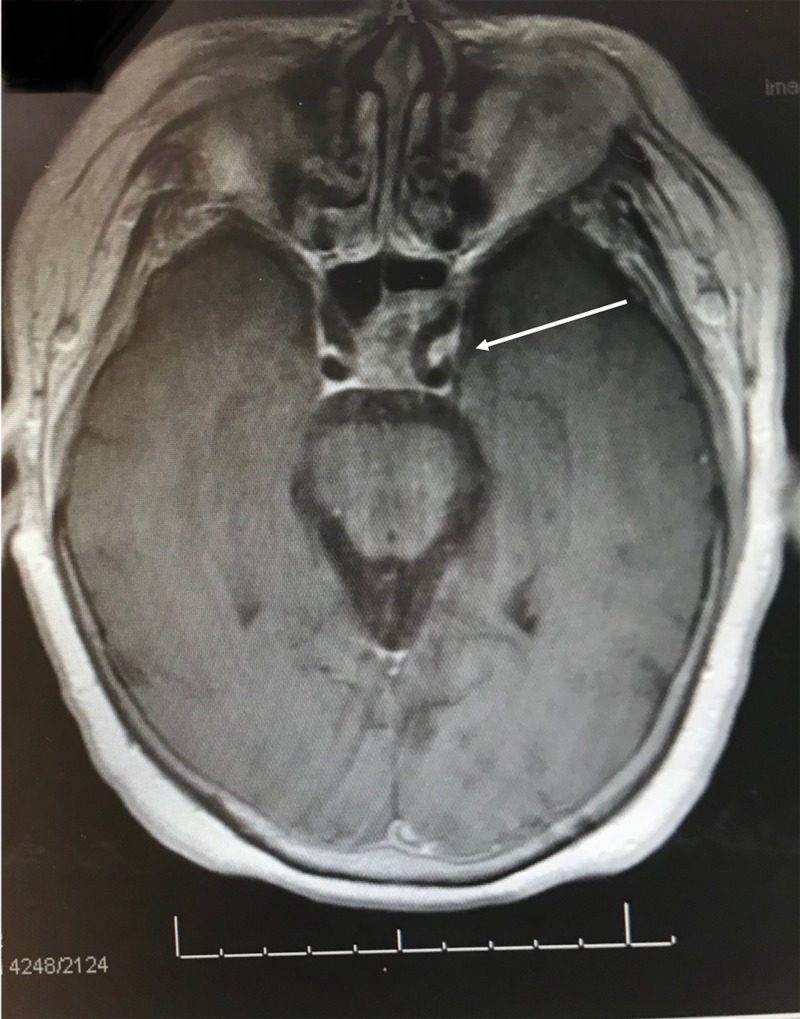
T1 post-contrast axial magnetic resonance imaging (MRI) showing heterogenous contrast enhancement of a clival mass that invades the left cavernous sinus, encasing the internal carotid artery
